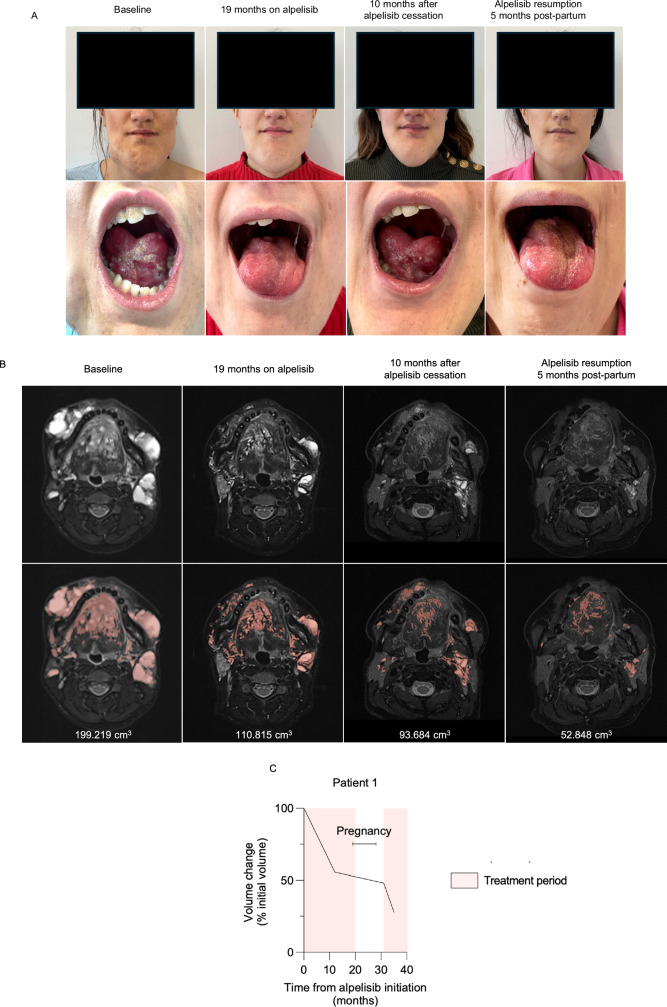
Patient 1 following alpelisib withdrawal and reintroduction. A Representative pictures of patient 1 over time. B Representative transversal MRI T2 fat sat sequences of patient 1 over time. C Magnetic resonance volumetric quantification of the malformation over time.
